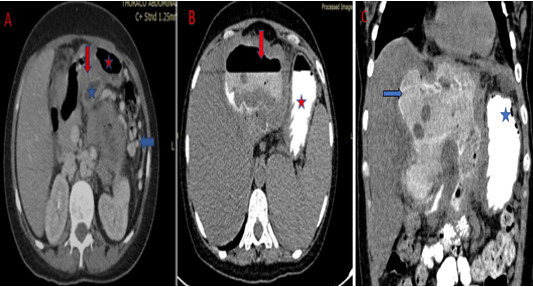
Abdominal CT scan in axial (A) sections with intravenous
